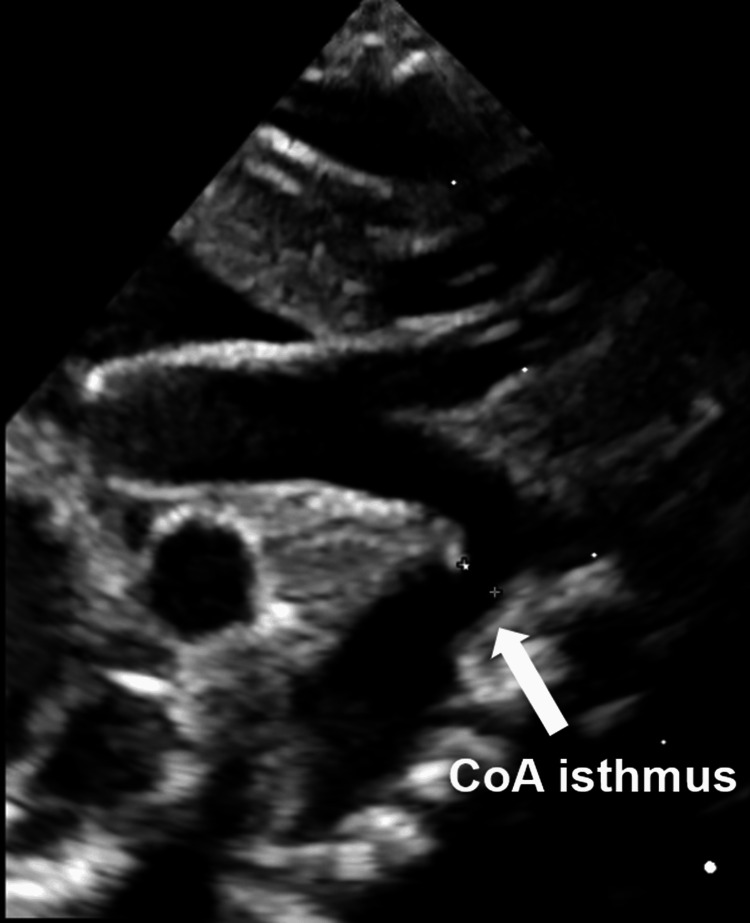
Transthoracic echocardiography demonstrating aortic isthmus narrowing consistent with coarctation Suprasternal notch long-axis view demonstrating discrete narrowing at the aortic isthmus (arrow), consistent with CoA. CoA: coarctation of the aorta
